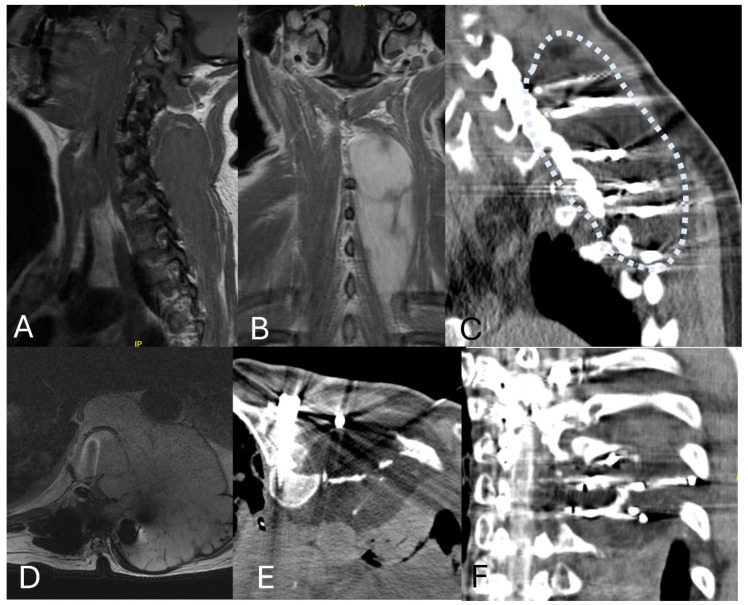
Cryoablation of large tumors: (A,B) Sagittal T1WI and Coronal Post Gadolinium T1WI demonstrate a 13 cm infiltrating paraspinal desmoid tumor in a 42-year-old female. (C) A total of 10 cryoablation probes were used, starting 1.5 cm from the superior margin and stacking probes vertically 2 cm apart. Sagittal CT demonstrates the ice ball with a 5 mm thermal margin around the lesion. (D) Axial T2WI demonstrates a large recurrent chondrosarcoma growing into the epidural space despite previous separation surgery and radiation. (E,F) After surgical removal of the epidural component through a smaller incision, the remaining tumor was cryoablated twice for 8 min. The patient lived 2.5 more years and died due to pulmonary metastasis burden.
